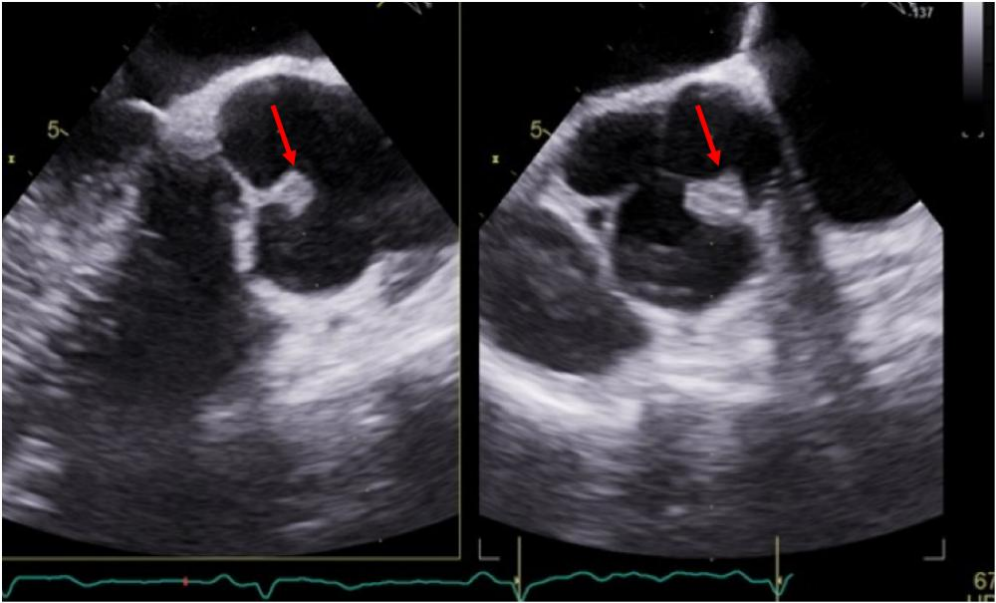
Transoesophageal echocardiography: pathological structure 8 × 12 mm fixed on the right/non-coronary commissure of the native tricuspid aortic valve (red stars).
